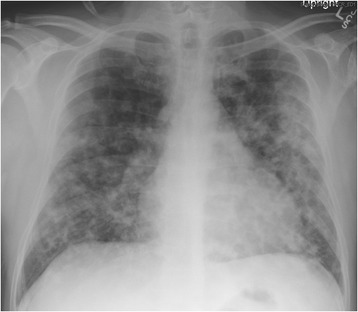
Upright AP Chest x-ray with bilateral, diffuse, airspace opacities was read as consistent with multilobar pneumonia possibly due to TB, MAI, aspergillosis or recurrent aspiration. The patient was ultimately determined to have pulmonary Kaposi’s sarcoma
